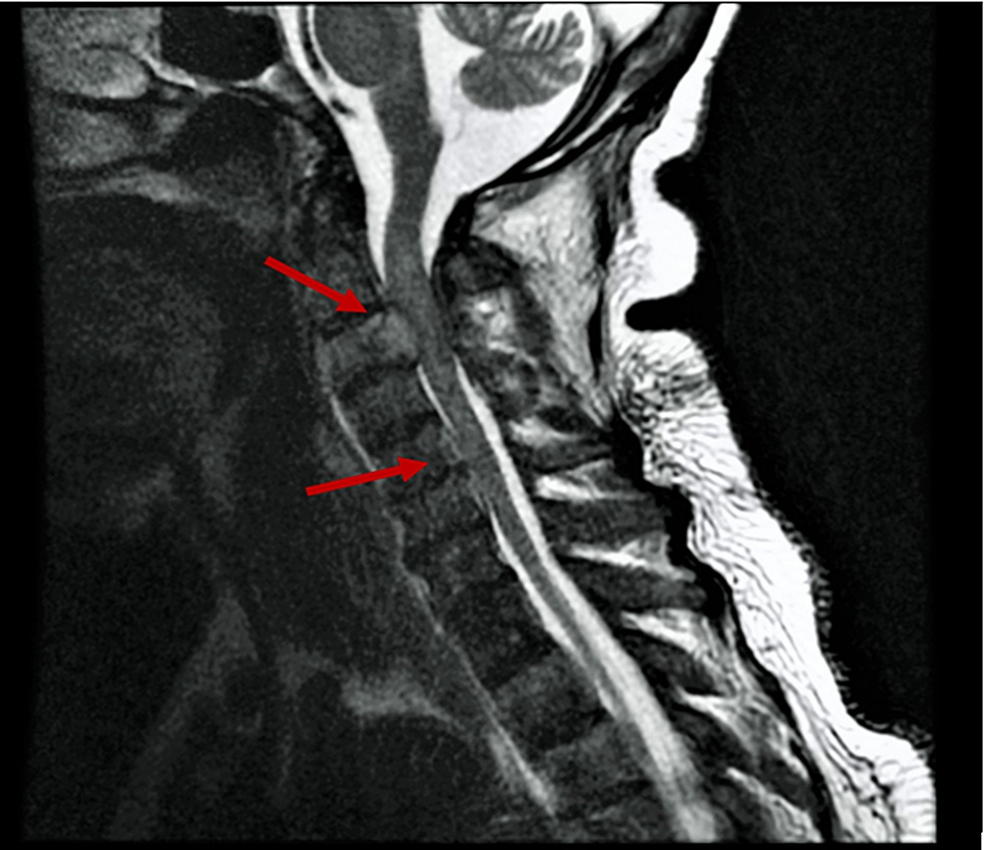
MRI of the spine. Spine MRI showing C3 lesion with posterior extension to the ventral and lateral epidural spaces encircling the spinal cord associated with cord compression, edema, and expansion (top arrow). There is an extensive osseous metastatic disease with cortical breakthrough along with compression from the C5-C6 vertebral bodies that effaces, but does not surpass the thecal sac at the C5-C6 level (bottom arrow).
